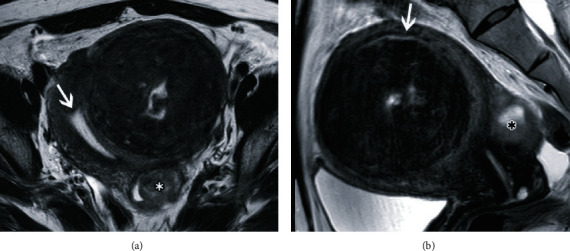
Magnetic resonance images before uterine artery embolization. (a) T2-weighted axial image demonstrates a large intramural fibroid. The central area has a high intensity, suggesting degeneration. The asterisk (∗) shows another degenerative uterine fibroid. (b) T2-weighted sagittal image demonstrates the endometrium (white arrow) compressed by the fibroid. The distance between the endometrium (white arrow) and the fibroid is very short. The asterisk (∗) shows another degenerative uterine fibroid.
